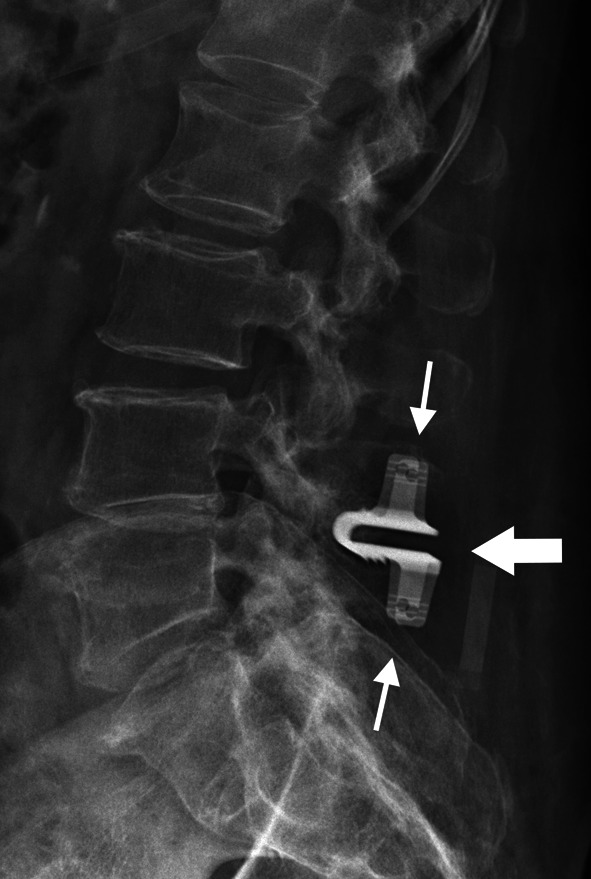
Dynamic stabilization system with interspinous spacer for intermittent neurogenic claudication. Lateral radiograph demonstrates the U-shaped device (arrow) placed into the interspinous space at the symptomatic level (L4-5). To maintain stability, the vertical keels are crimped onto the spinous processes (thin arrow). This procedure requires resection of both interspinous and supraspinous ligaments. A common complication in such procedures is spinous process resorption along the implant close to the fixation site. There are other devices available for the same proposal with less invasive approaches
